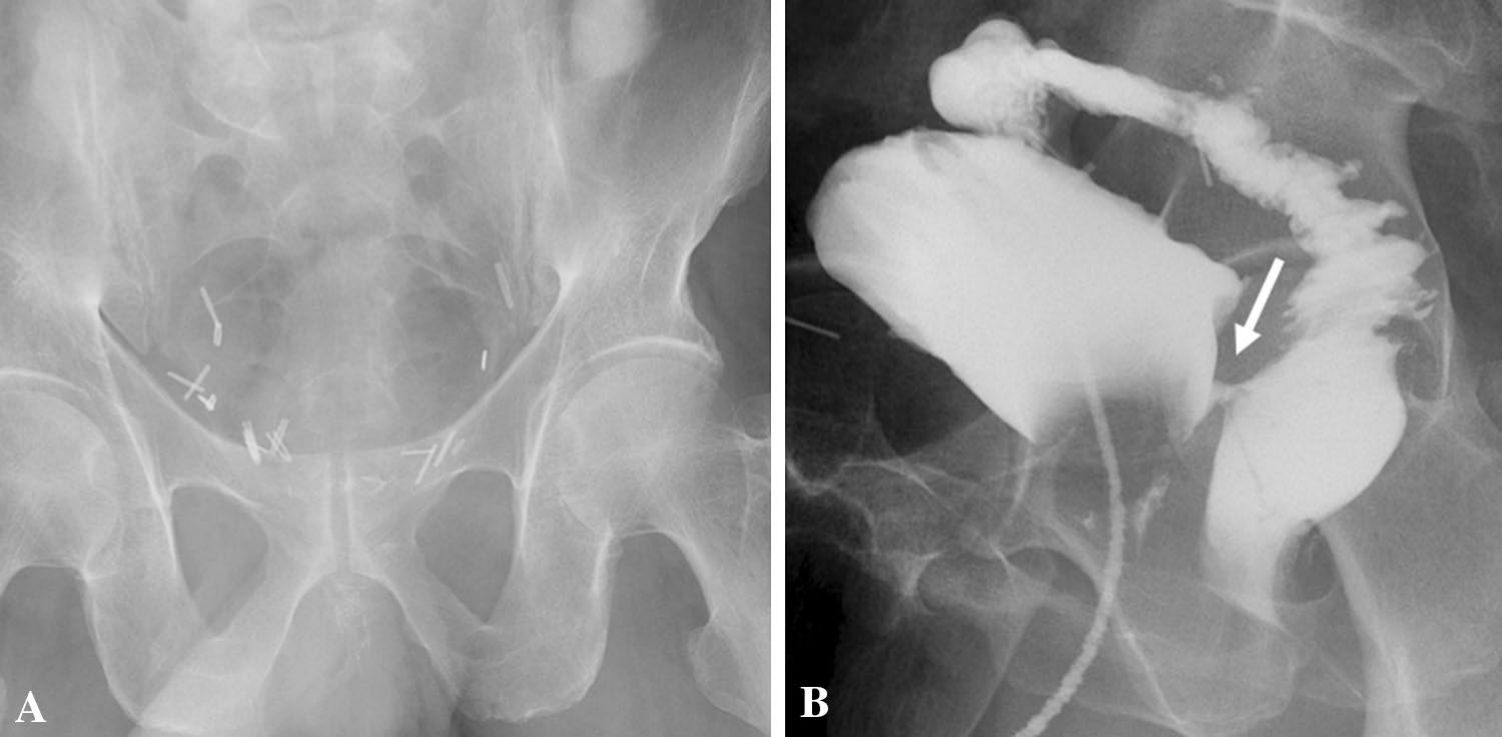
Iatrogenic posterior urethral injury. Plain radiograph (a) and voiding cystourethrogram (b) in an elderly gentleman with a prostato-rectal fistula (arrow), post-abdominoperineal resection and radical prostatectomy
